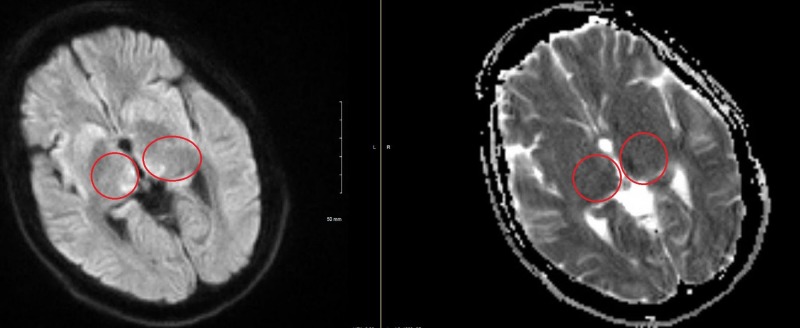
DWI MRI of brain shows cytotoxic edema on bilateral thalamic regions with corresponding ADC changes significantly on the left when compared to right (red circles) Abbreviations: DWI = diffusion restriction imaging; MRI = magnetic resonance imaging; ADC = apparent diffusion coefficient
